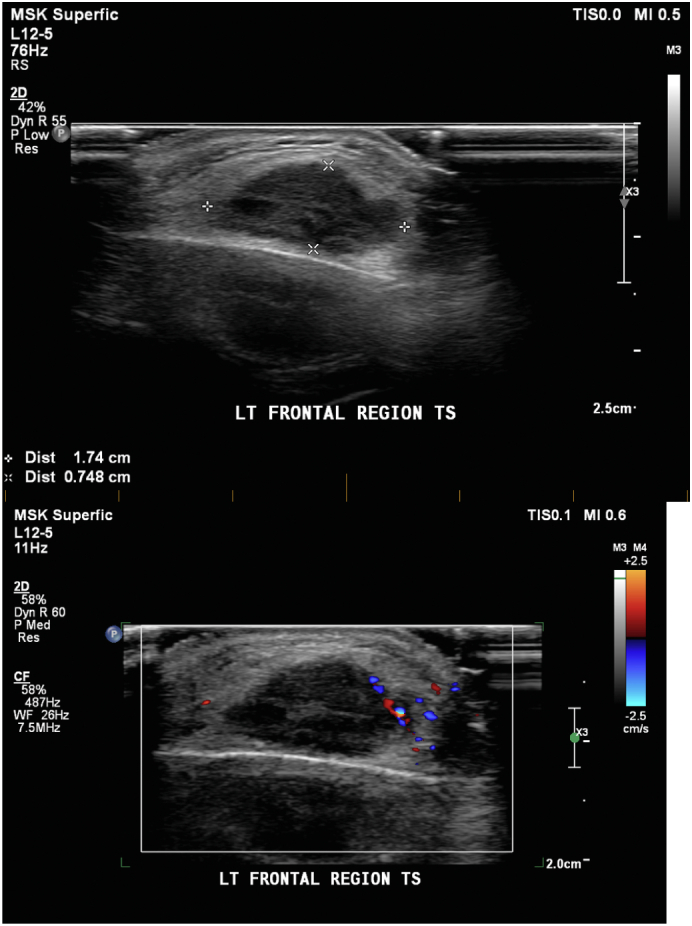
Ultrasound images of the patient's ECM pre operatively showing a hypoechoic lesion measuring 17x7x21mm, with no significant vascularity.
